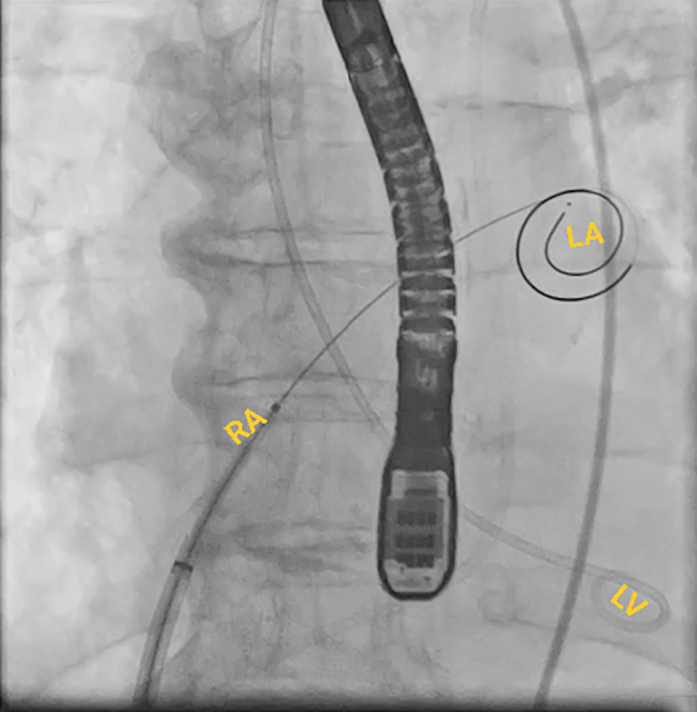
Fluoroscopic Image of the Procedure Fluoroscopic image of a Safari wire positioned in the LA through an atrial septal puncture. A transesophageal echocardiography probe is present in the center of the image. A pigtail is placed across the aortic valve. Abbreviations as in Figure 2.
